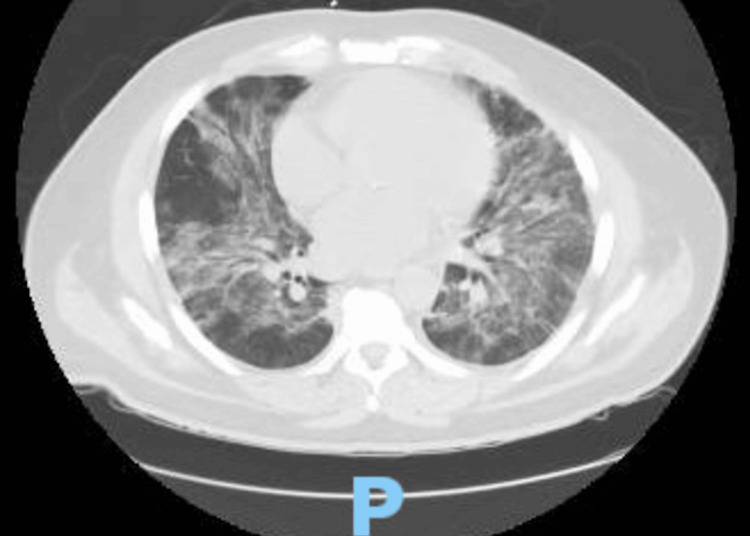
Repeat CT chest performed on hospital day seven to evaluate the patient’s respiratory status, which demonstrated increased bilateral ground glass and consolidative opacities, concerning worsening pneumonia.
